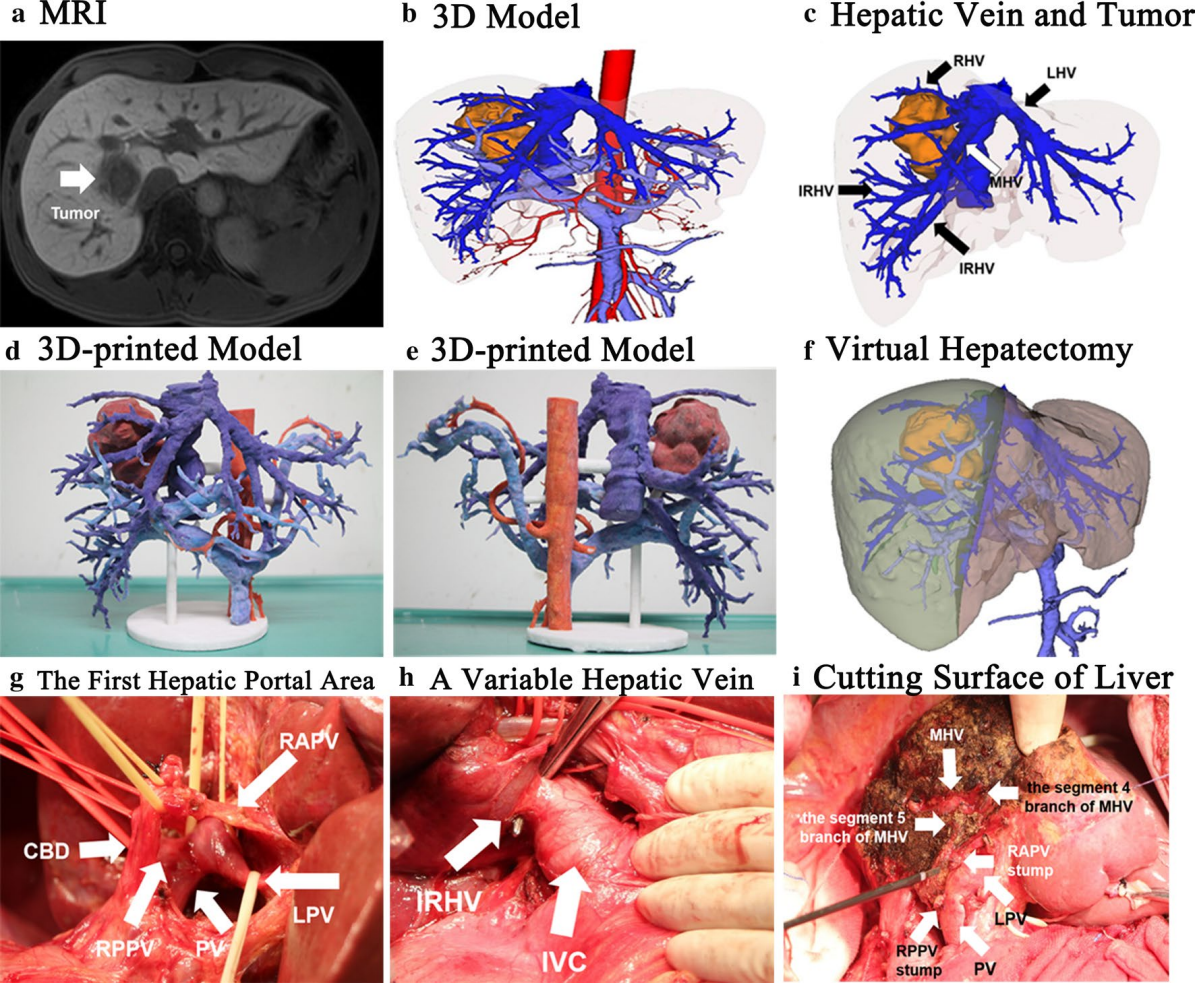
Complicated hepatectomy guided by 3D printing technology. A Type III variation of portal vein, inferior right hepatic vein (IRHV) flowing into IVC. a MRI showed that the tumor was located in the right-anterior liver; b CT-based 3D model; c 3D model showed the relationship between tumor and variant hepatic vein; d 3D printed model (anterior view); e 3D printed model (posterior view); f virtual hepatectomy; g portal hepatis structures; h variant IRHV flowing into IVC; i after tumor resection, the course of branches of middle hepatic vein on segments 4 or 5 and the transected ends of RAPV and RPPV can be seen. *LHV* left hepatic vein, *MHV* middle hepatic vein, *RHV* right hepatic vein, *IRHV* inferior right hepatic vein, *PV* portal vein, *LPV* left-portal vein, *RAPV* right-anterior portal vein, *RPPV* right posterior portal vein, *CBD* common bile duct, *IVC* inferior vena cava
